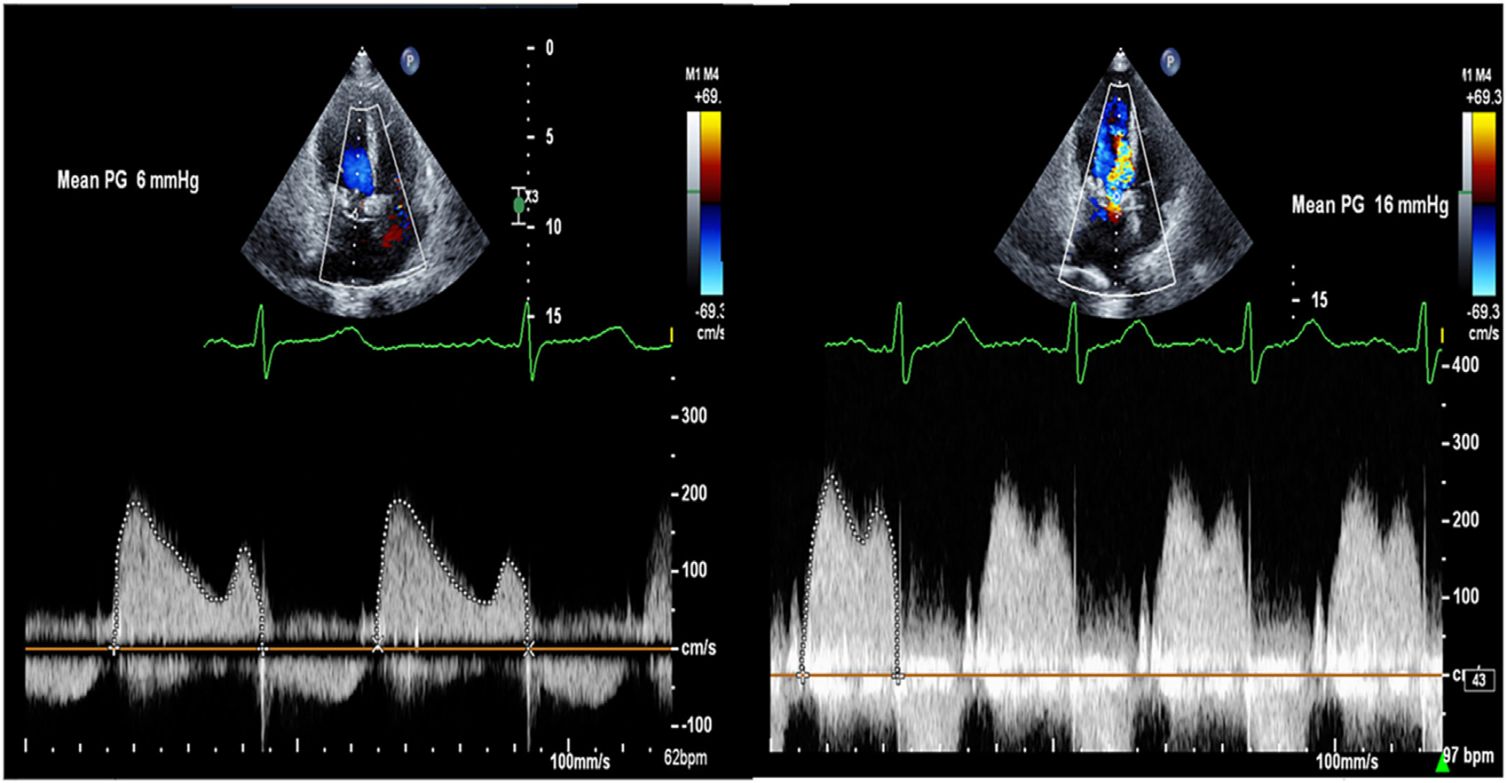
Preoperative stress test echocardiogram demonstrating mean mitral valve gradient of 6 mm Hg at rest and 16 mm Hg at peak stress. (PG, prosthetic valve gradient.)
